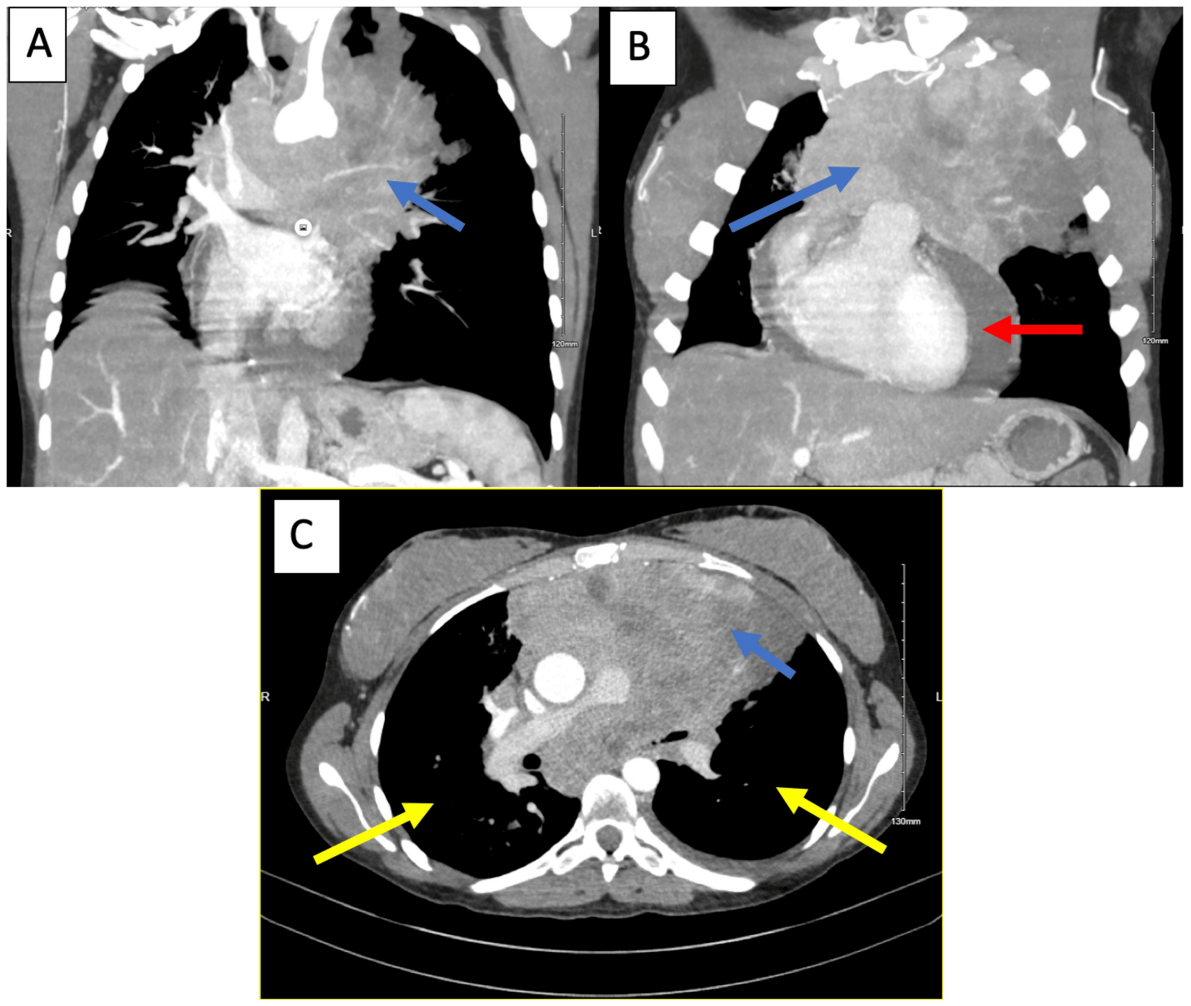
Chest CT. The initial chest CT demonstrates (A, B) >15 cm anterior mediastinal mass with extension into bilateral hilum and posterior mediastinum, moderate to severe pericardial effusion (blue arrows), (B) left pleural effusion (red arrow), and (C) bilateral ground-glass opacities (yellow arrows).
